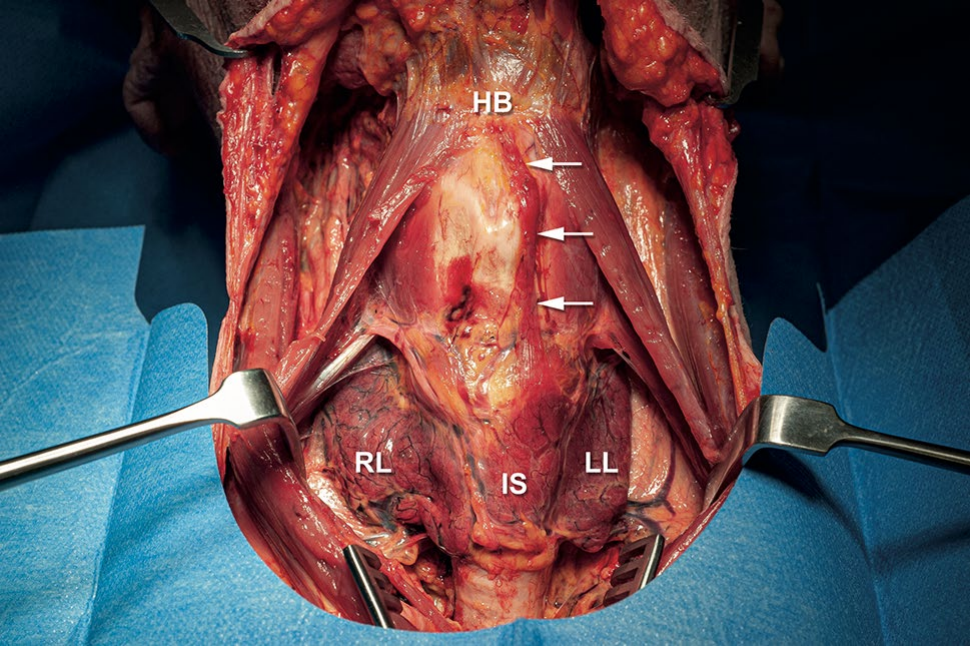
The same 80-year-old male cadaver after dissection. A left-sided PL (arrows) with connection to the hyoid bone that was previously detected with ultrasound was exposed. *RL* right lobe of the thyroid gland, *LL* left lobe of the thyroid gland, *IS* thyroid gland isthmus, *HB* hyoid bone
